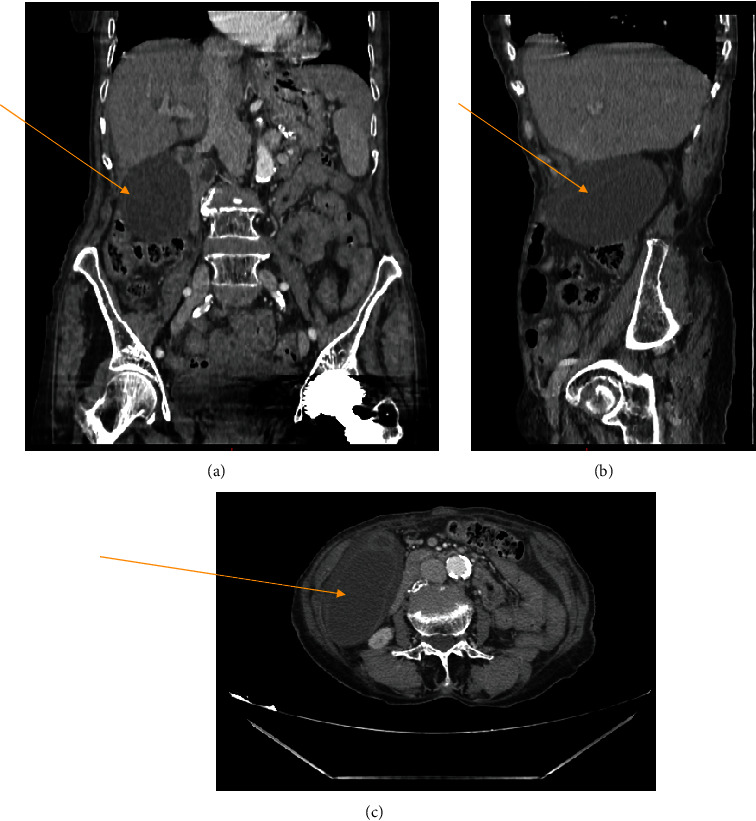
Multislice CT scan of abdominal and pelvic with IV contrast media in 1.25 mm slices thickness.
